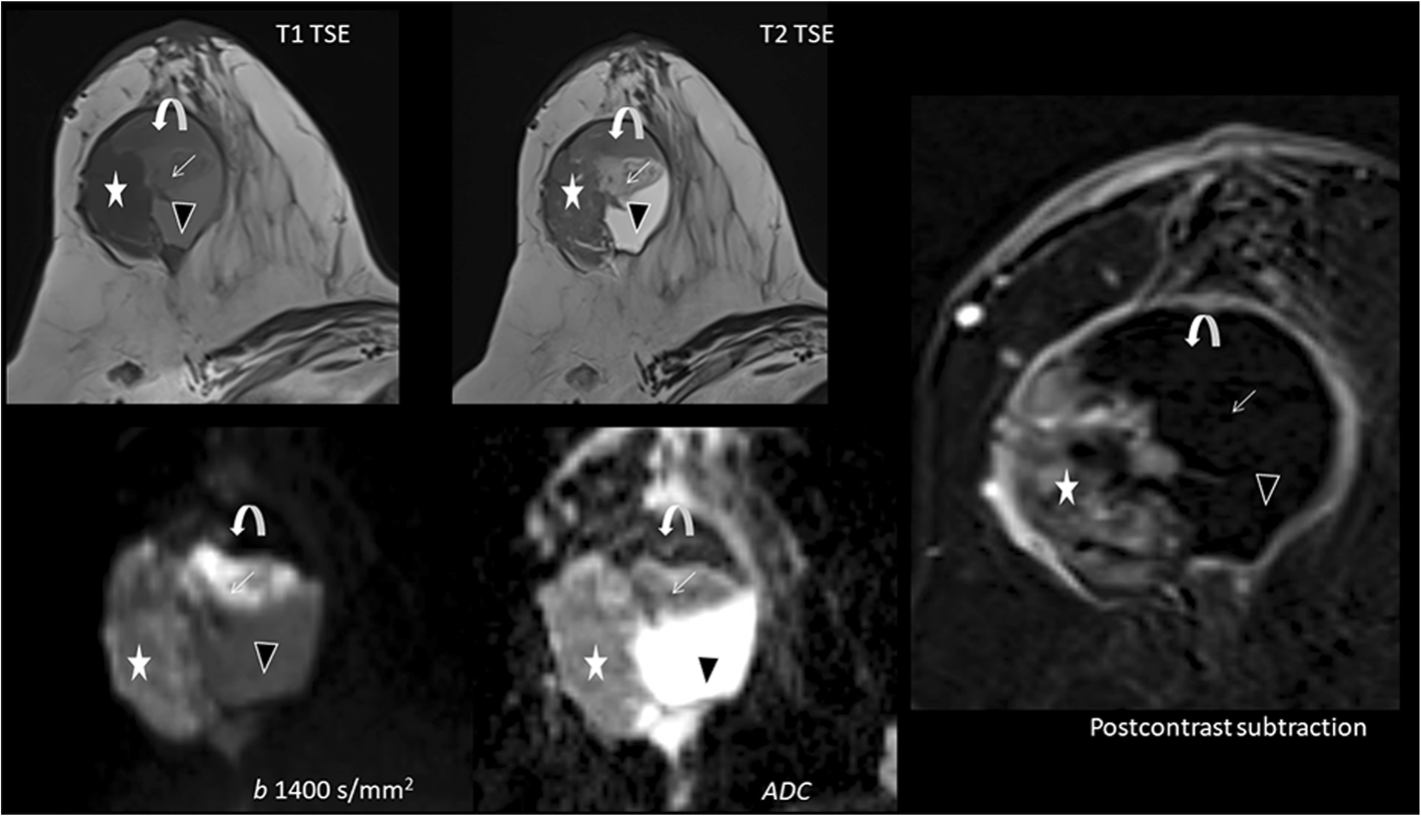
Papillary tumor of the breast. A well-bordered complex cystic mass with the solid portion (star) restricting diffusion. The lesion shows three different appearances of hemorrhage. Appearances of hemorrhagic contents of the tumor depend on several factors. The content located at dependent part (curved arrow) is T1 and T2 hyperintense, and may be superimposed by the susceptibility artifacts due to degradation products and being very hypointense on both DW-MRI and ADC map. The hemorrhagic contents located at the central part of the lesion (arrow) restricts diffusion because bright on DW-MRI and dark on ADC map. This can be due to intact red blood cell membranes with intracellular oxyhemoglobin, deoxyhemoglobin, or methemoglobin and clot retraction. Lastly, the content marked black arrowhead shows no restriction can be explained by disintegration products of the bleeding and more chronic stages
